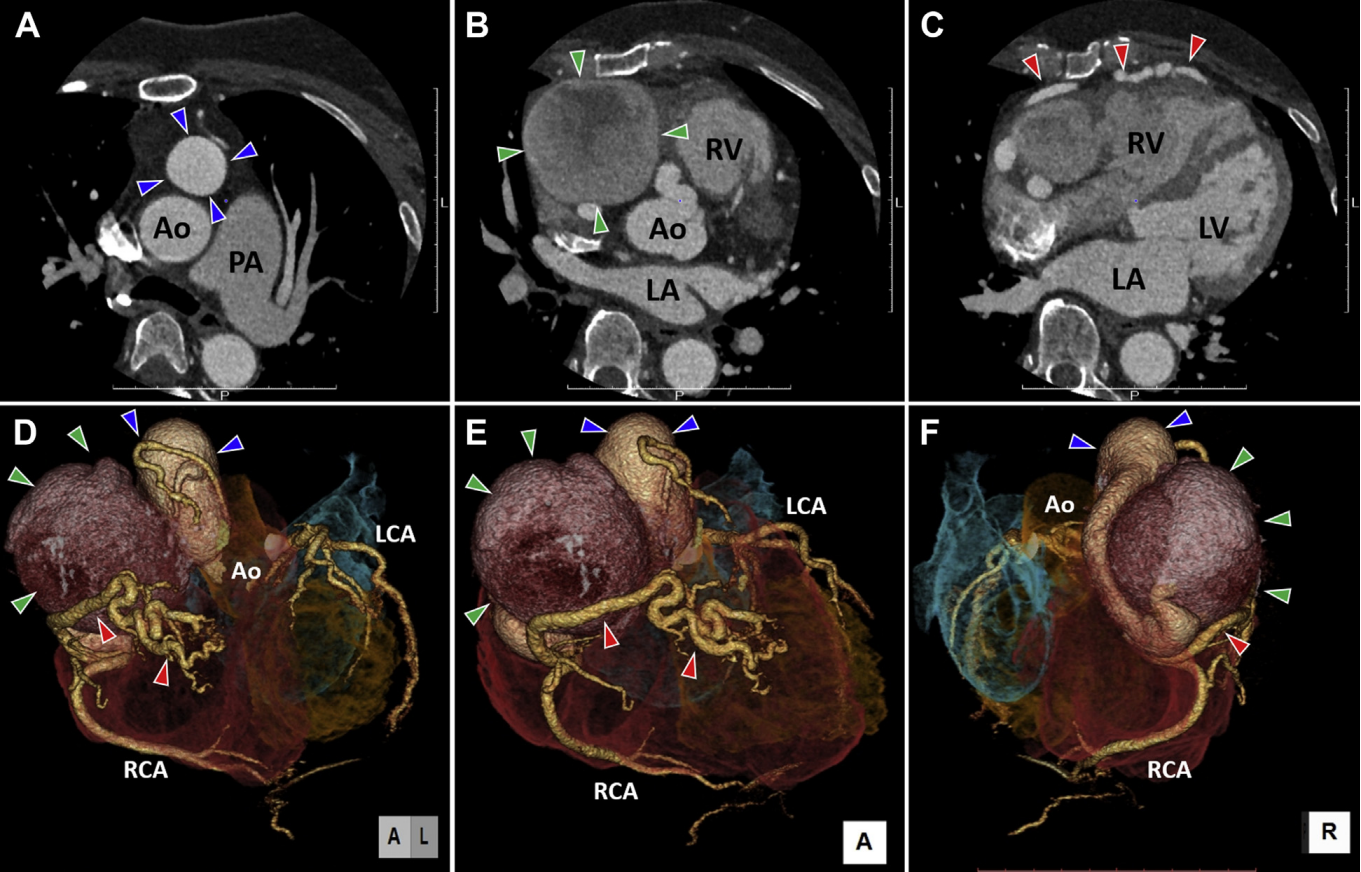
Pre-Operative Enhanced Computed Tomography Imaging (A) The right coronary aneurysm (diameter 28 mm; blue arrowheads) anterior to the aorta (Ao) (axial image). (B) Giant pseudoaneurysm (maximum size 63 mm; green arrowheads) on the right side of the heart. (C) Abnormally dilated fistulas (red arrowheads) branching off the right coronary artery (RCA) and communicating with the right ventricle (RV). (D to F) 3-dimensional computed tomography imaging showing the anatomical relation between the pseudoaneurysm (green arrowheads), right coronary aneurysm (blue arrowheads), and coronary artery fistula (red arrowheads). RCA just distal to the coronary artery fistula is healthy. D is the left anterior view; E is the anterior view; and F is the right view. Note that inflows of the pseudoaneurysm, coronary fistula, and healthy RCA were branched off from the right coronary aneurysm. LA = left atrium; LCA = left coronary artery; LV = left ventricle; PA = pulmonary artery.
